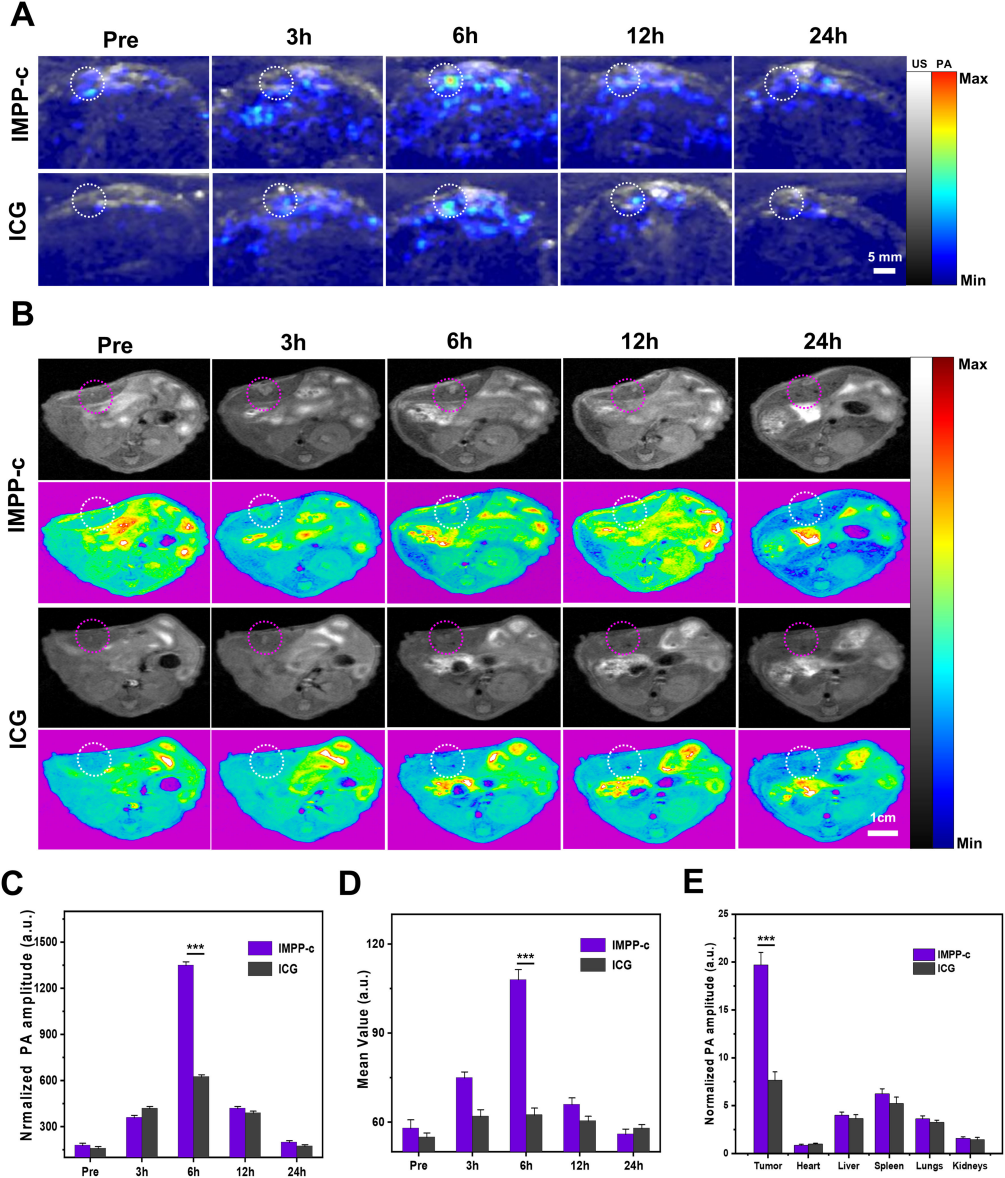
In vivo* PA/MR imaging of orthotopic tumors. (A)
